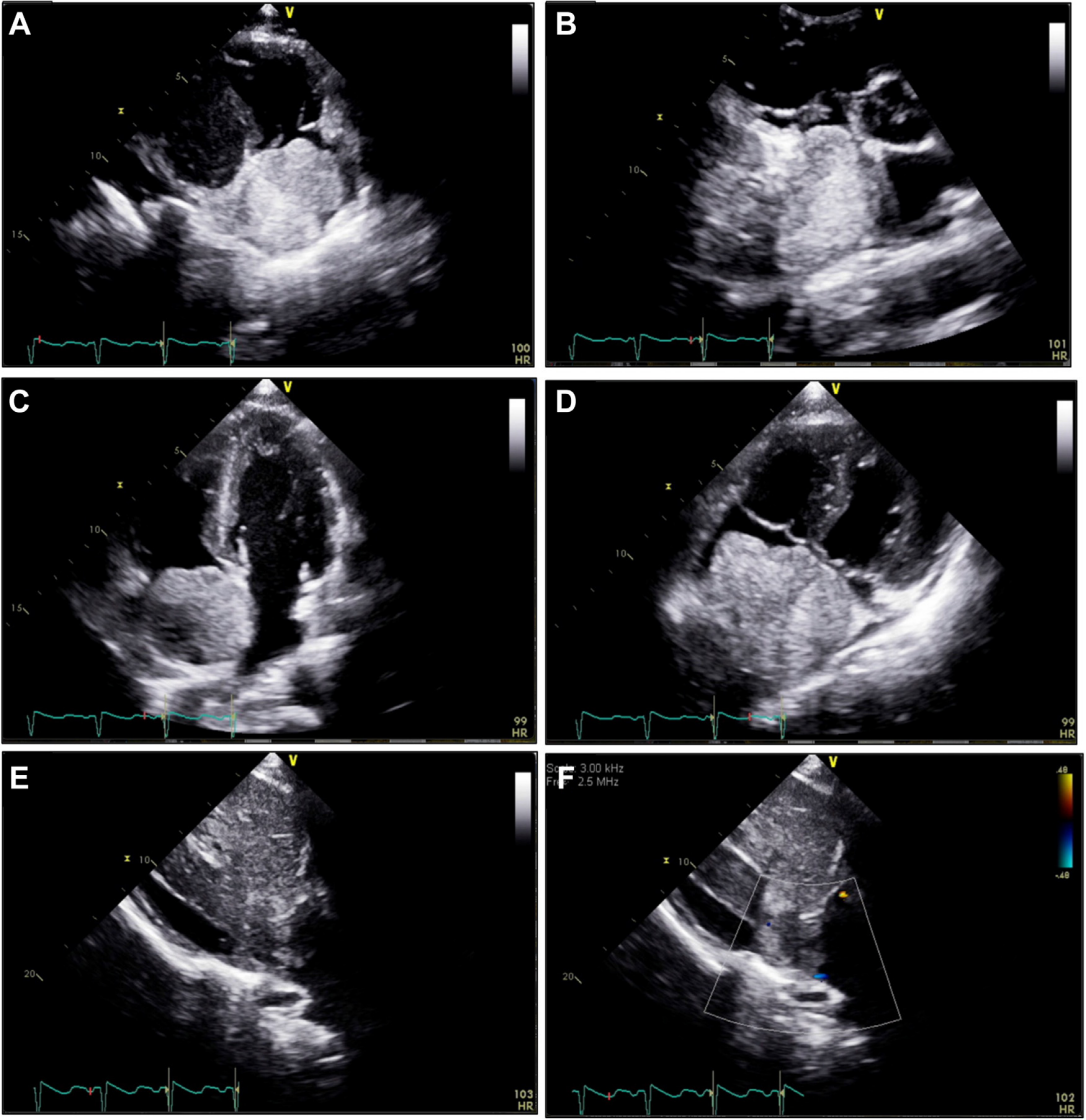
Hepatocellular Carcinoma in the Right Atrium (A) Right ventricular inflow view demonstrating the right atrial mass occupying the entire right atrium. (B) Parasternal short-axis view with a focus on the right atrium demonstrating the mass. (C) Apical 4-chamber view both on axis with the left ventricle and (D) off axis with a focus on the right ventricle demonstrating the right atrial mass with possible attachment to the interatrial septum. (E) In the subcostal view, the right atrial mass appeared to have originated from the inferior vena cava, and (F) color Doppler demonstrated minimal flow to the right atrium because of the mass, causing obstruction.
